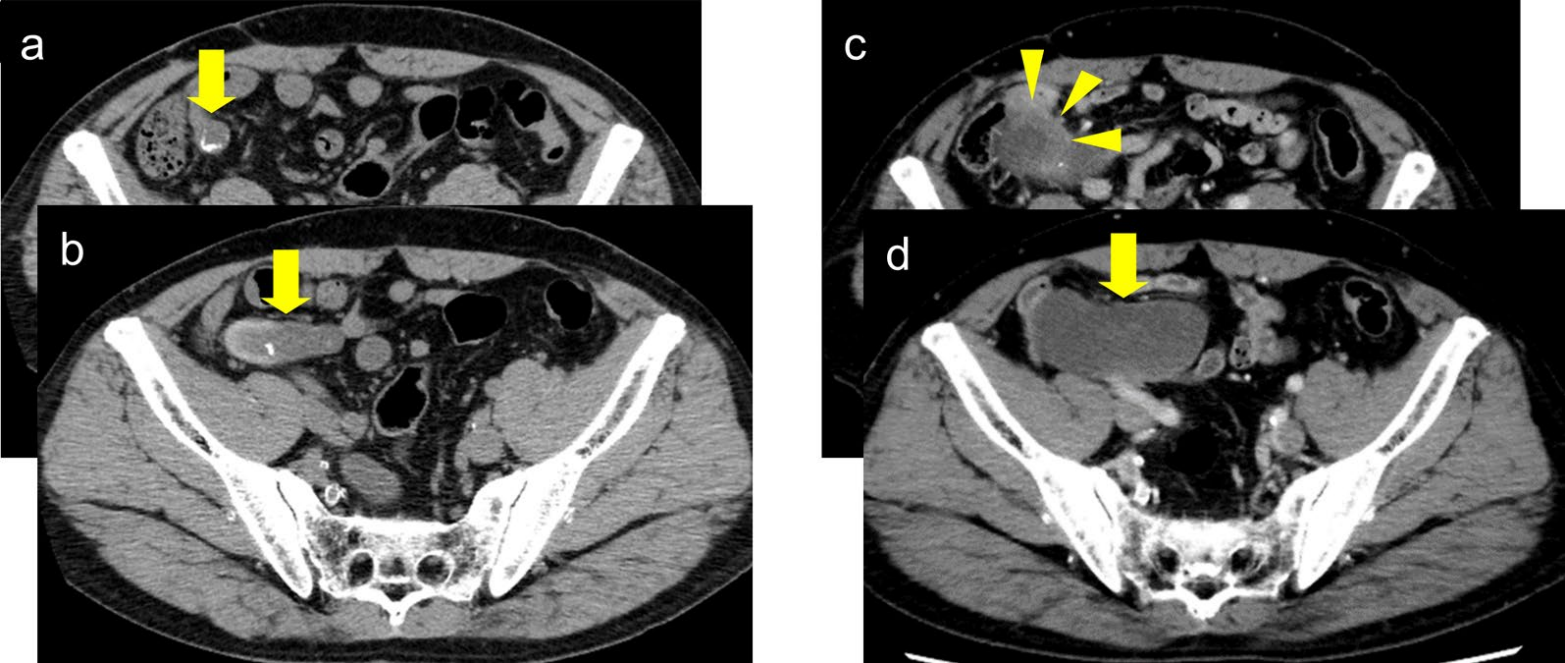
Changes of CT findings overtime in case 2. a, b A cystic lesion (55*22mm) adjacent to the ileocecum was identified 19 years after appendectomy. c, d The cystic lesion was enlarged (85*35mm) with irregular wall thickening (arrowheads) 21 years after appendectomy
